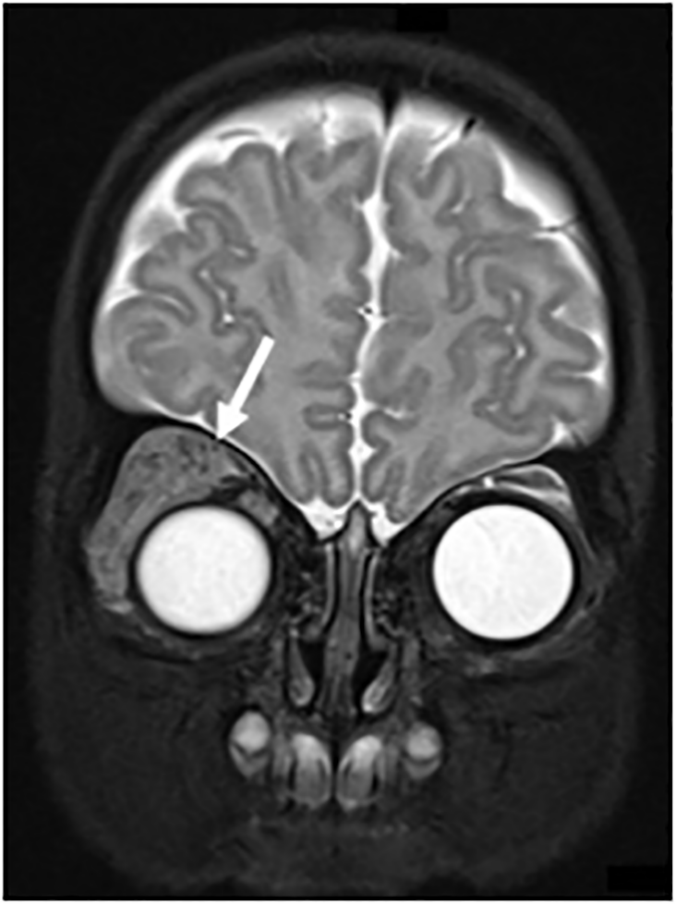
Magnetic resonance imaging of brain and orbits demonstrating signal
